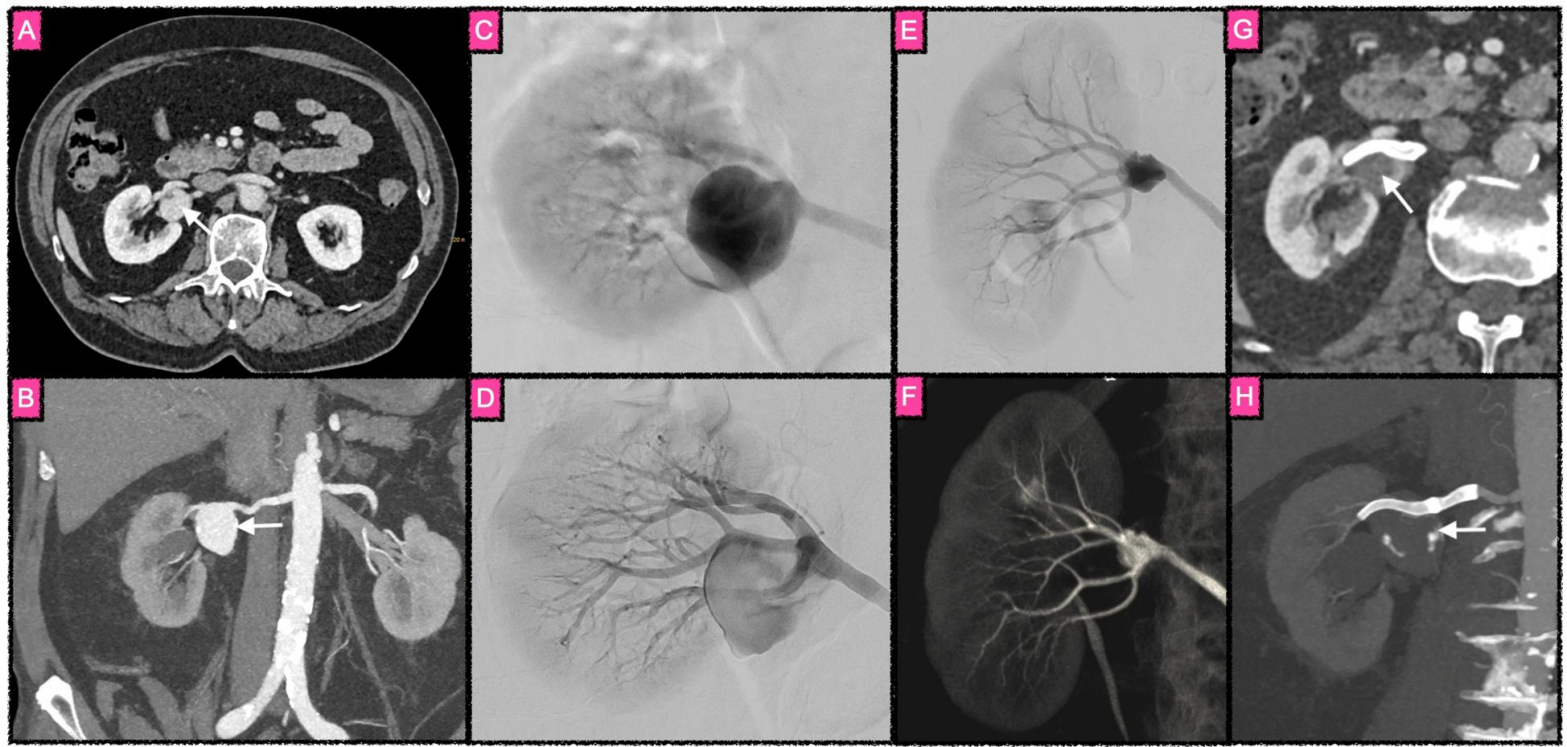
Course of FDS treatment of an aneurysm involving the right renal artery trifurcation. (A) and (B) Axial and coronal computed tomography angiographic images of the large renal artery aneurysm (white arrows) prior to treatment. (C) Superselective catheter angiogram of the right renal artery prior FDS implantation. The broad neck of the aneurysm is not clearly visualized in the images prior to treatment. (D) After implantation of two slightly overlapping FDS, with the distal landing zone in the superior branch of the renal artery trifurcation and the proximal landing zone in the renal artery main stem, the aneurysm is less opacified. The neck is clearly visible and contrast agent stagnation is depicted until the late parenchymal phase, corresponding to O'Kelly–Marotta scale grade A2. (E) Superselective control DSA 6 months after treatment with a reduced volume of the perfused aneurysm sac and contrast agent stagnation until the venous phase, corresponding to O'Kelly–Marotta scale grade B3. (F) Corresponding image of a 3D rotational angiography at the six months follow-up. (G) and (H) Axial and coronal computed tomography angiographic images of the aneurysm (white arrows) twelve months after treatment with a small residually perfused remnant close to the FDS construct, corresponding to O'Kelly–Marotta C3. Covered segmental arteries remain perfused, and there is no renal infarct visible.
